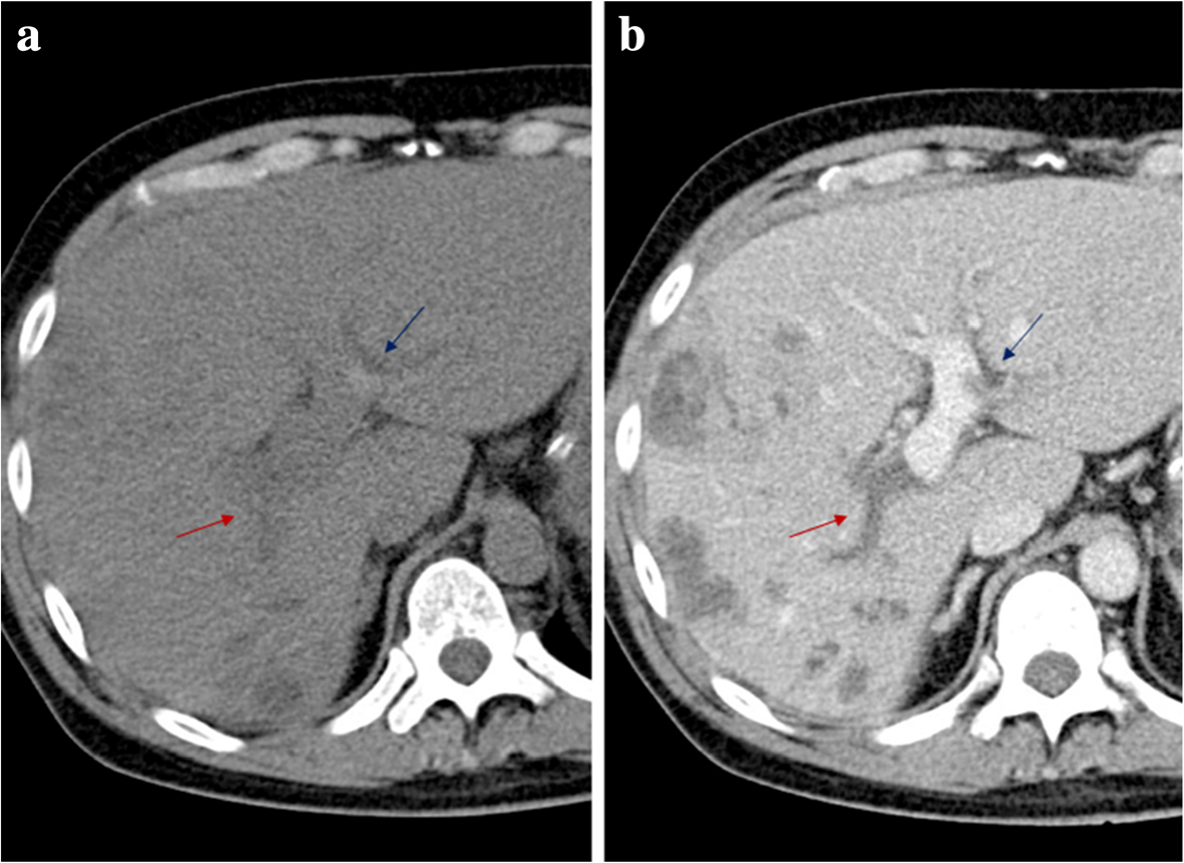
Acute vs. subacute/chronic PV thrombosis. a Plain CT axial image shows high-attenuation content within a left PV branch (blue arrow)—acute thrombus. b Portal venous phase CT axial image clearly reveals an additional low-attenuation filling defect in the right PV, extending into its right posterior branch (red arrow)—subacute/chronic thrombus
